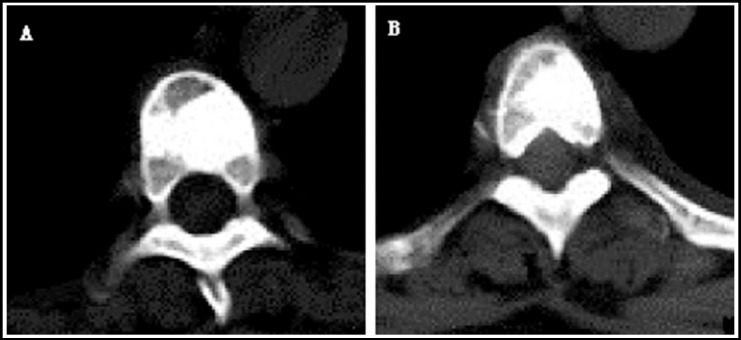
Postoperative CT scanning demonstrated good distribution of bone cement in T4 (A) and T7 (B) and pains relieved.
